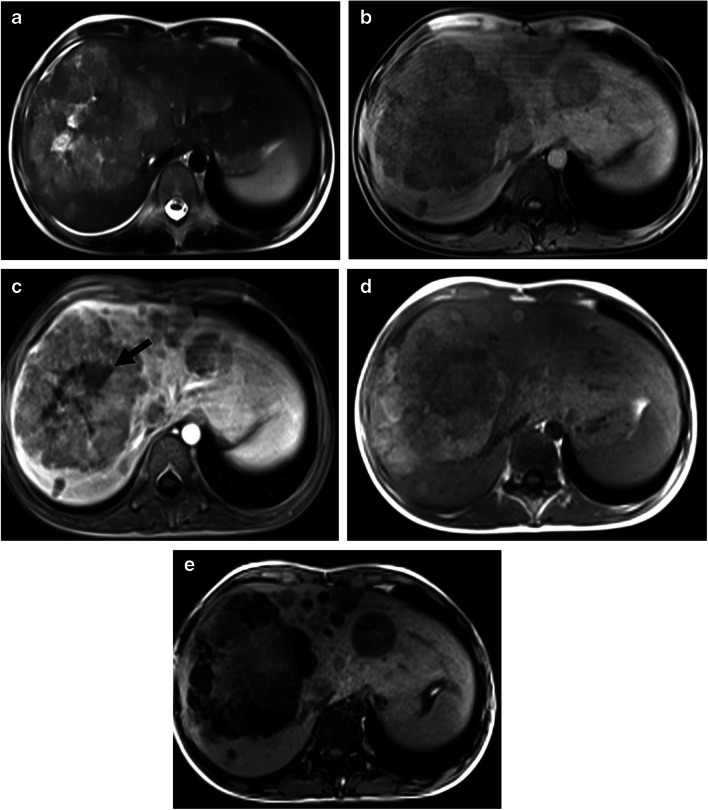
Hepatoblastoma in a 13-year-old boy. a–c Axial T2-weighted (a), and precontrast (b) and postcontrast (c) T1-weighted images show multifocal tumor with necrosis (*arrow*) and heterogenous enhancement. d, e Dual-echo in-phase (d) and out-of-phase (e) GRE T1-weighted images show signal loss on out-of-phase due to intralesional fat
